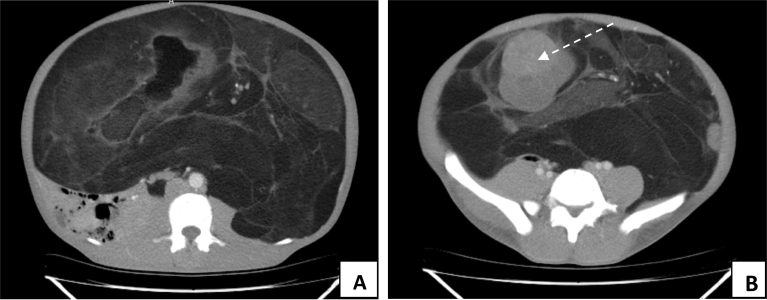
A: Computed tomography imaging showing a giant homogenous mass, mainly consisting of fatty tissue measuring 30 cm*36 cm*39 cm with thin septa, pushing the peritoneal contents such as bowel loops posteriorly and with internal enhancing nodular components), B: Thin arrow depicting large enhancing component).
